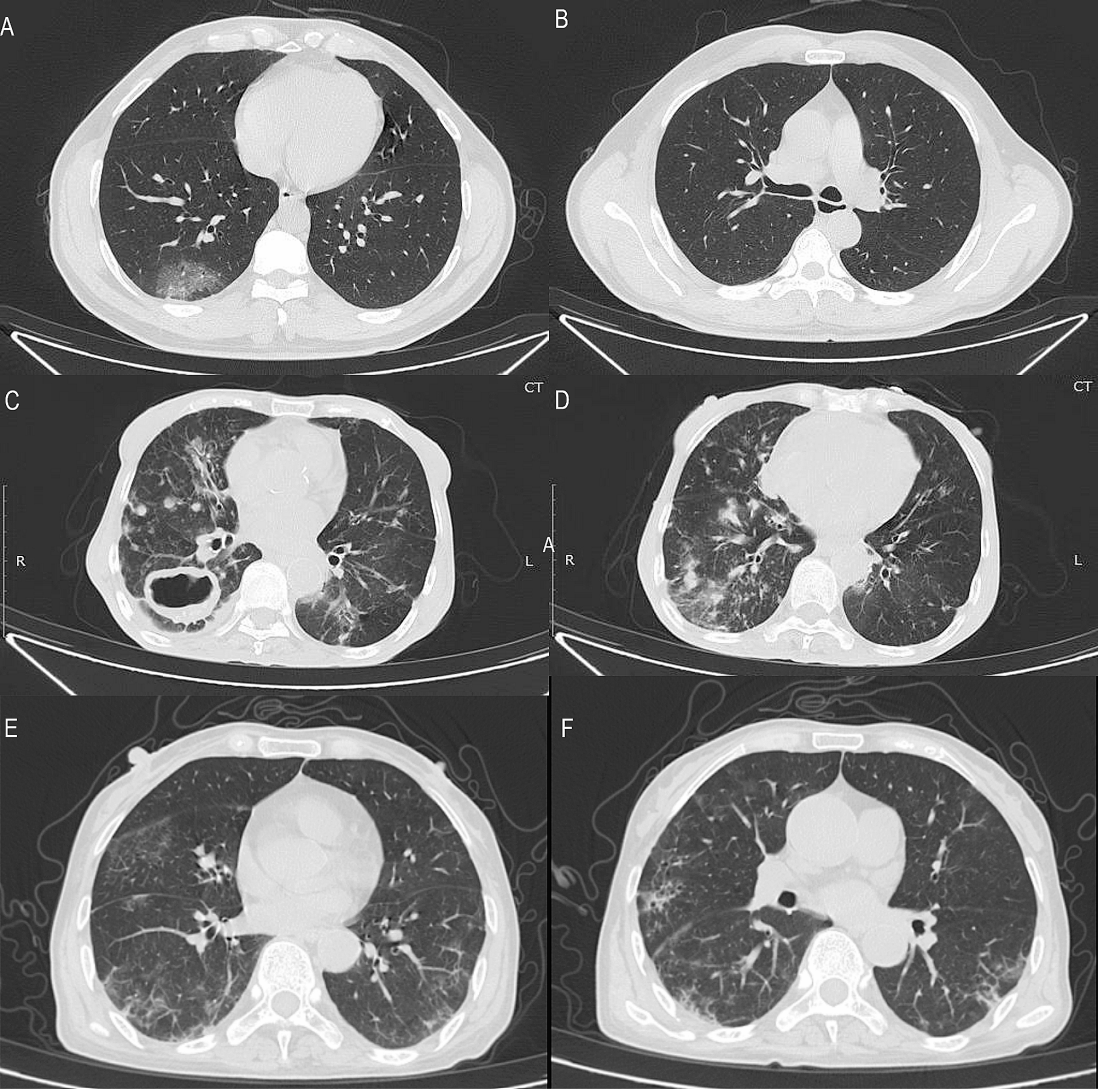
Representative chest CT images. (A and B) Transverse chest CT image of a 48-year-old man infected with Delta only showing patchy ground-glass opacities in the inferior lobe of the right lung five days after symptom onset. (C and D) Transverse chest CT images from a 66-year-old woman coinfected with *Mtb* showing tuberculous cavities and bilateral multiple patchy shadows on day six after symptom onset. (E and F) Transverse chest CT images from a 58-year-old woman coinfected with HIV showed multiple patchy, streaky ground-glass opacities in both lungs on day two after symptom onset.
